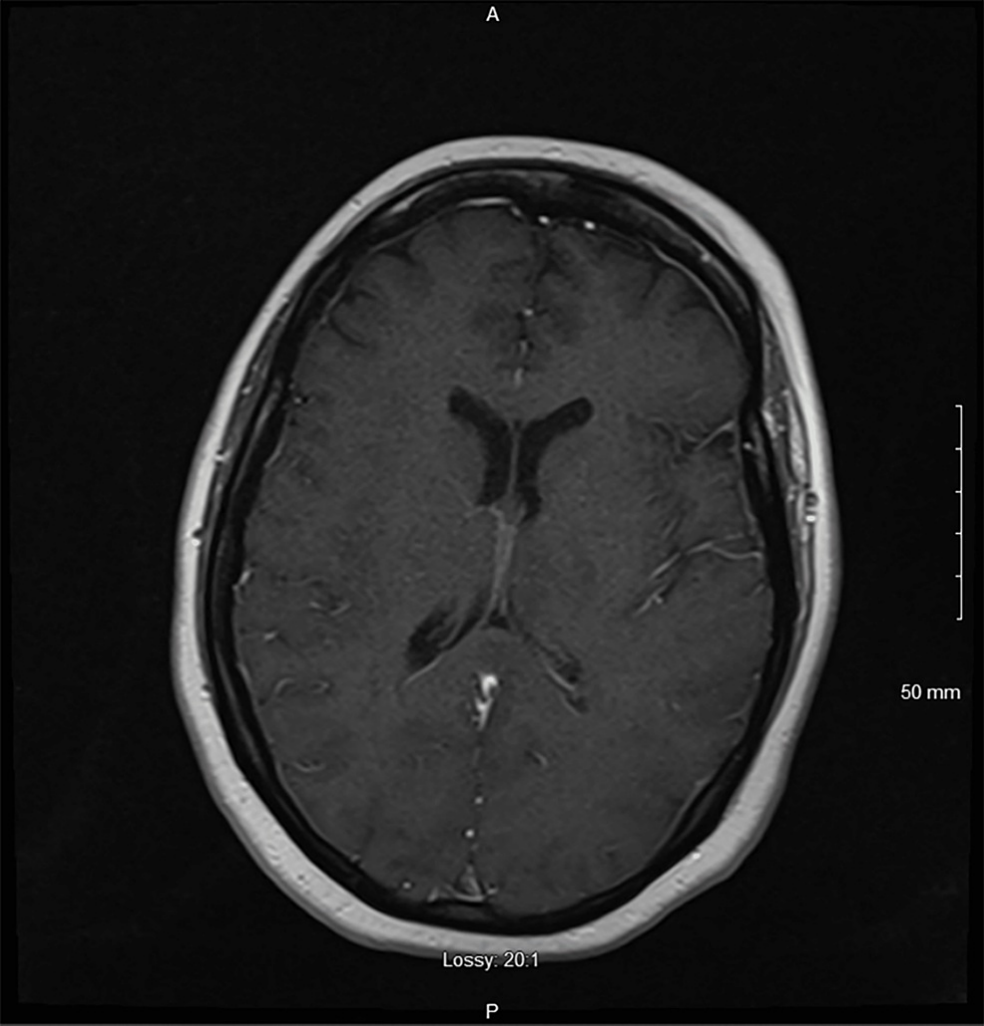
MRI of the brain showed no acute cortical abnormalities.
